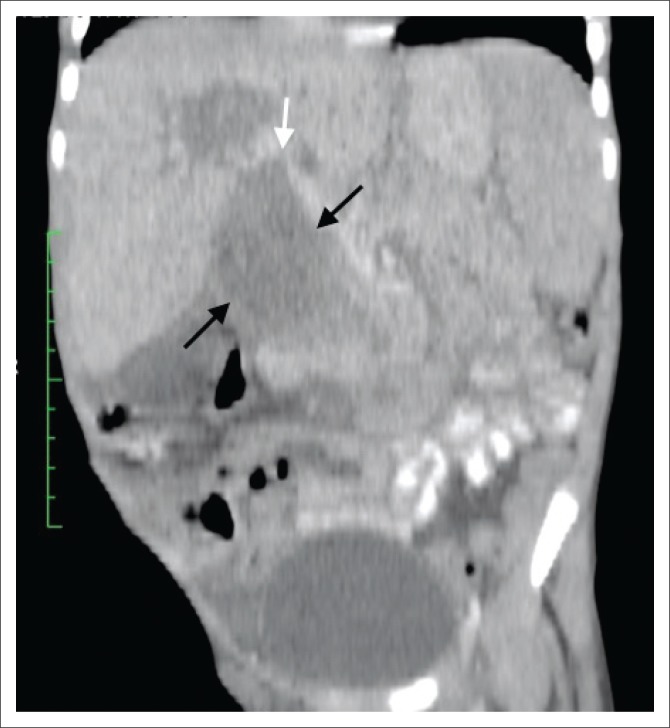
Coronal post contrast computed tomography image demonstrating a heterogeneous mass within the common bile duct (black arrows) with the superior aspect not separable from the adjacent porta hepatis or proximal central bile ducts (white arrow).
